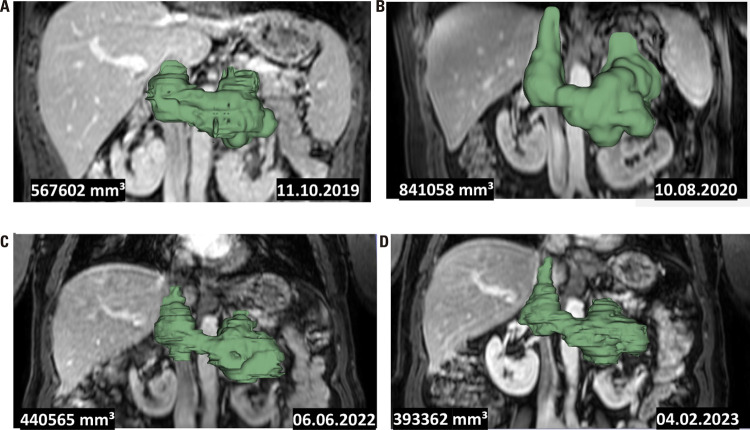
Abdominal computed tomography images analyzed using the software 3D Slicer, Version 5.0.2 (https://www.slicer.org/) to depict the tumor volume regression over time. The images depict the tumor volume (in mm3) at the time of diagnosis (A), immediately before stereotactic ablative radiotherapy (SABR) (B), and 18 months (C) and 27 months (D) after SABR treatment.
